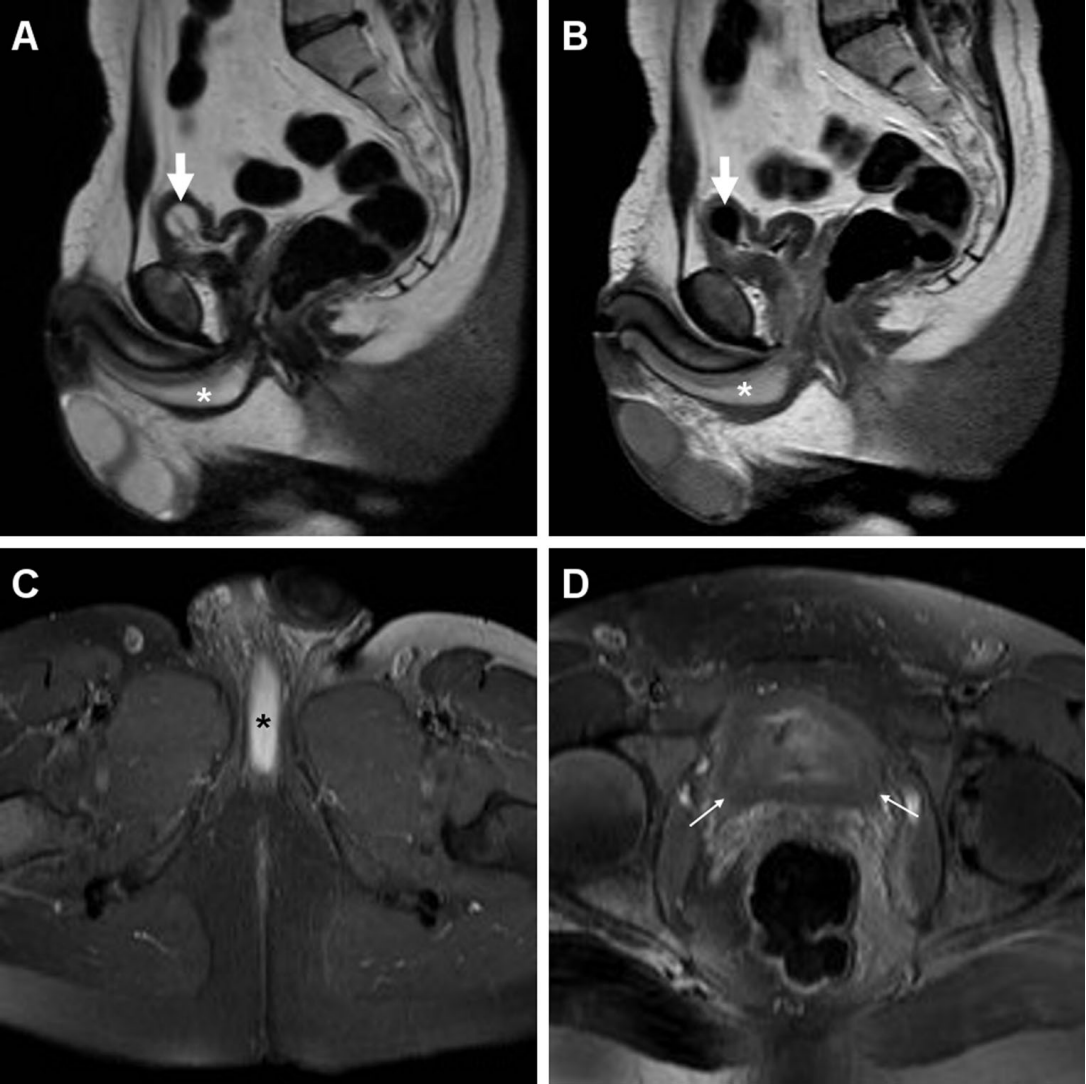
Acute uncomplicated urethritis in a 30-year-old man with neurogenic bladder treated by intermittent self-catheterisation. Physical examination revealed induration and tenderness of the corpus spongiosum and purulent urethral secretions. Unenhanced T2-weighted MRI images (a) revealed a diffuse, uniform hypersignal in the corpus spongiosum (*) with corresponding intense homogeneous enhancement on post-gadolinium T1- weighted sequences (b, c). The infection did not appear to interrupt the tunica dartos or Buck’s fascia, and did not involve the corpora cavernosa, scrotum or ischioanal spaces. Note Foley catheter in place (*thick arrows*). The patient successfully recovered with temporary suprapubic catheter and intravenous and topical antibiotics [Partially reproduced with permission from Ref. [62]]
